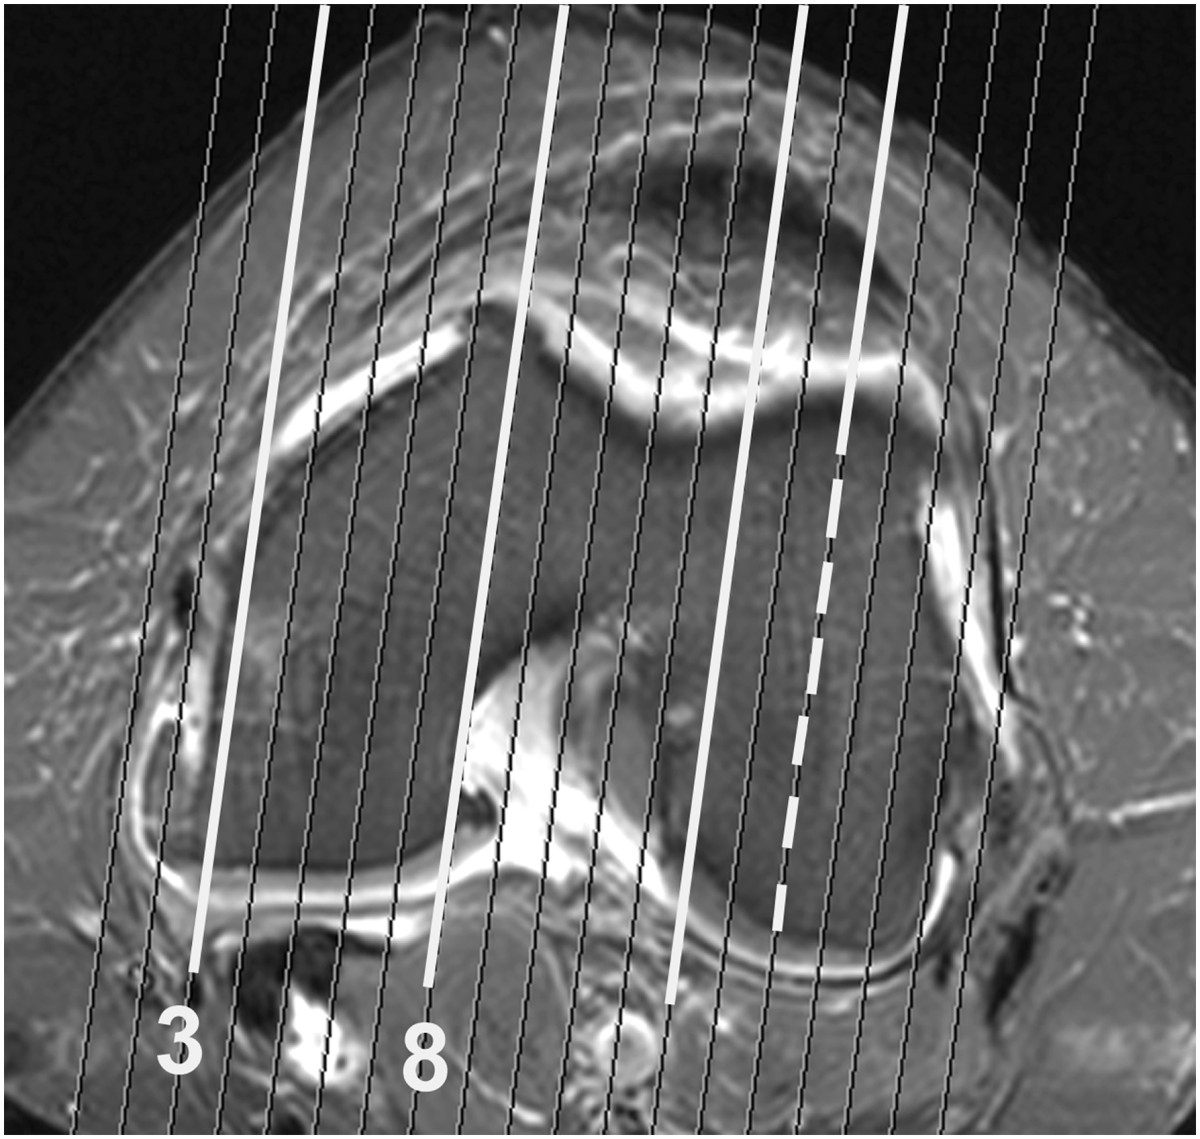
Trans-axial post-contrast T1FS MR image of the femoral condyles showing the direction of sectioning perpendicular to a line joining the dorsal aspects of the medial and lateral femoral condyle with a width of 4.4 mm. Optimally, the central slice of the lateral femoral condyle (broken line) is used for estimating the reference values, See Figure 2. Sections adjacent to the inter-condylar area and at the joint margins may contain partial volume information from soft tissue structures (white lines). Four sections of the medial femoral condyle (between section 3 and 8) are available for the measurements of bone marrow lesions (BMLs). Note that the medial condyle decreases ventrally resulting in decreasing bone marrow area on sagittal images medially.
